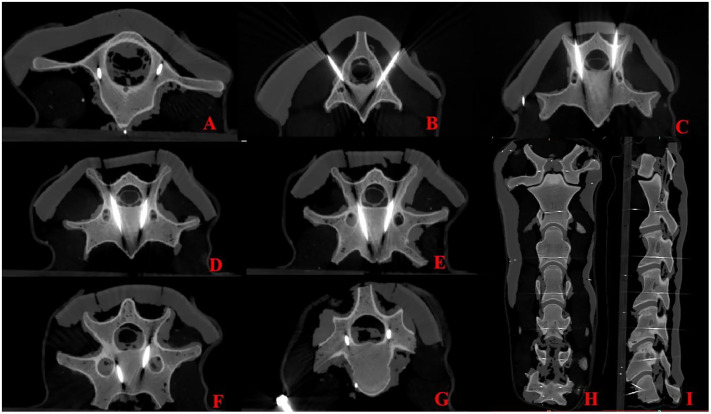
Postoperative CT Images of the Needle-Included Skeleton and Kirschner Wire. A-G Postoperative vertebrae (C1-C7) and Kirschner wire in the horizontal plane; H Vertebrae and Kirschner wire in the coronal plane.; I Vertebrae and Kirschner wire in the sagittal plane.
